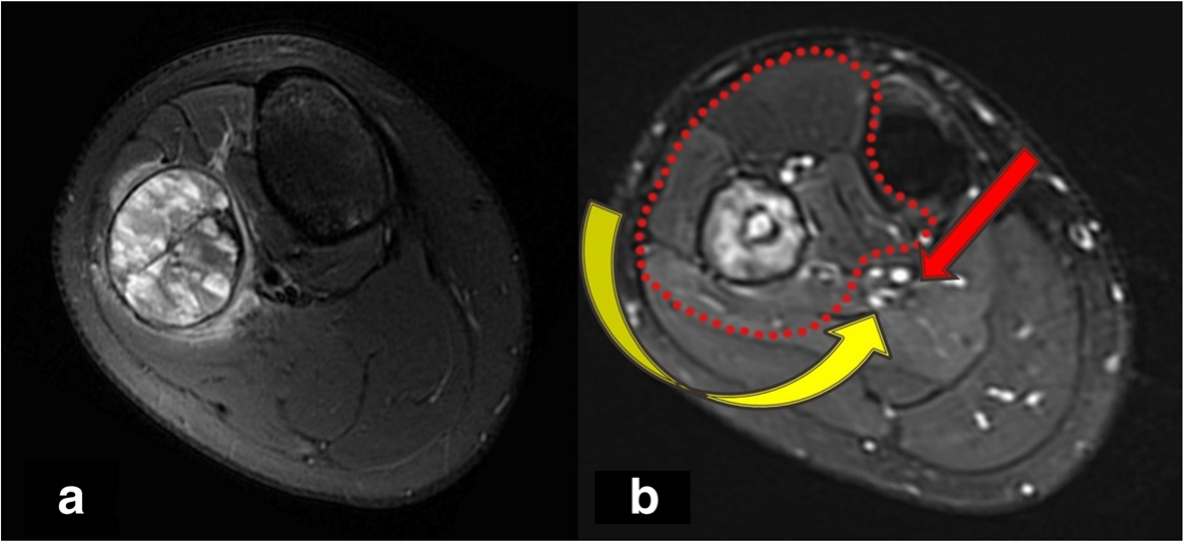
a MRI (T2 weight imaging) revealed that the medial-posterior aspect of the proximal fibula was involved by tumor combined with the tibial vessels and nerve. b After adjuvant chemotherapy, good result was observed with obvious ossified shell formation and tumor shrink. However, in terms of handling the vessel and nerve, it is difficult to deal from one lateral approach (yellow arrow); we recommended the double approach (adding medial approach, red arrow)
